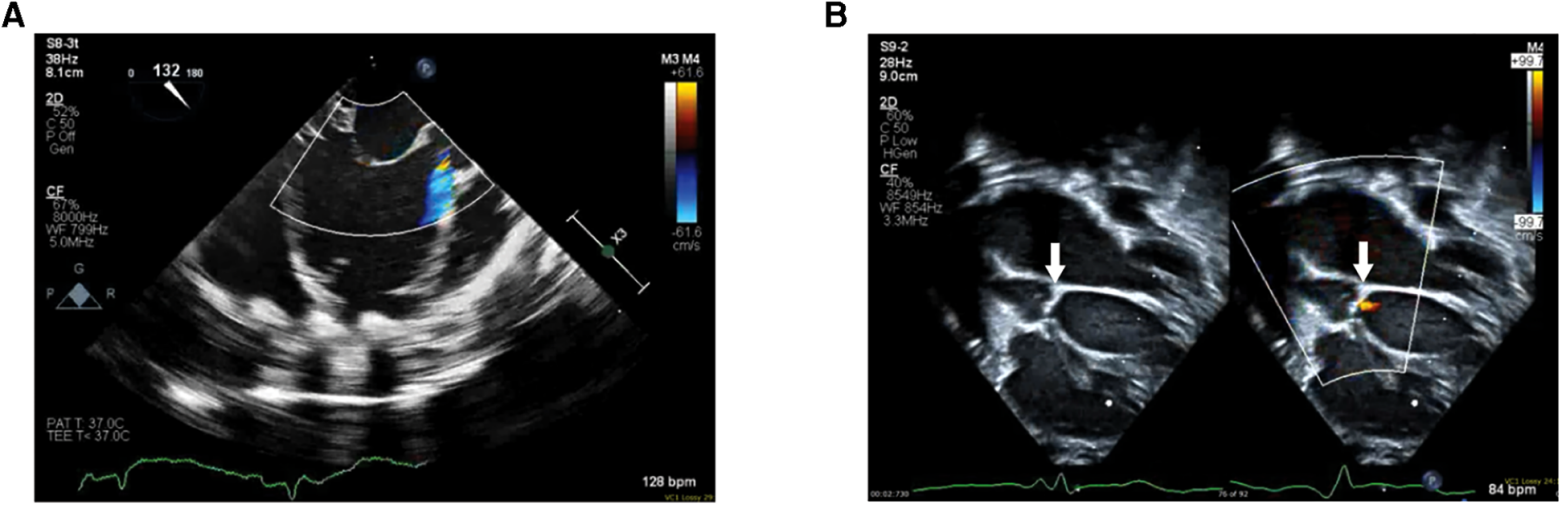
(A) Pre-VAD implantation echocardiogram with color doppler mapping demonstrating significant AI. (B) Post-VAD implantation 2D echocardiogram (left) with color-doppler comparison (right) demonstrating the patch placed at the ventriculoaortic junction (white arrows) and trivial patch margin leak.
